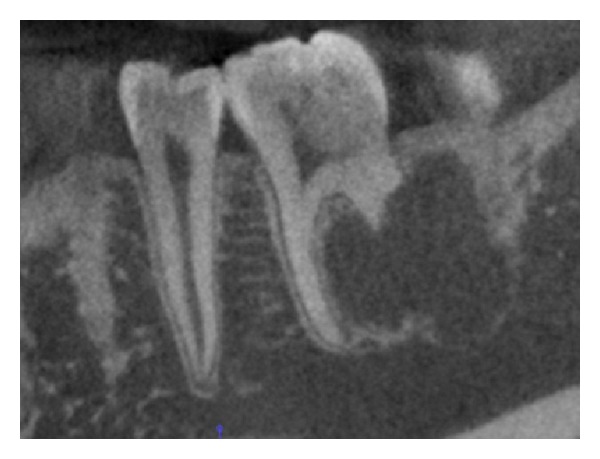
Panoramic radiograph demonstrating unilocular radiolucency with erosion of the distal root of tooth #19.
